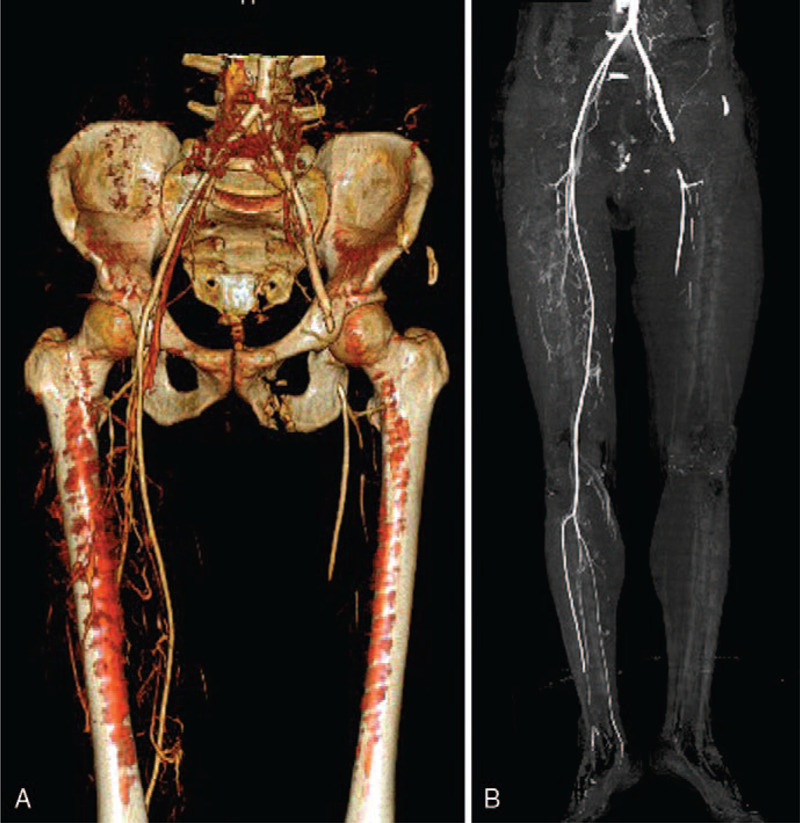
Case 2, CTA showed multiple fractures of the pelvic and a total occlusion of the distal end of the left external iliac artery and the left femoral artery. CTA = computed tomography angiography.
